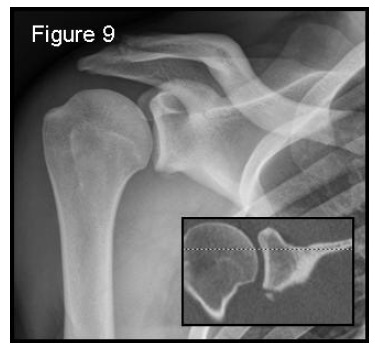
Grashey view: a true antero-posterior X-ray view in the scapular plane. 55x51mm (150 x 150 DPI).
